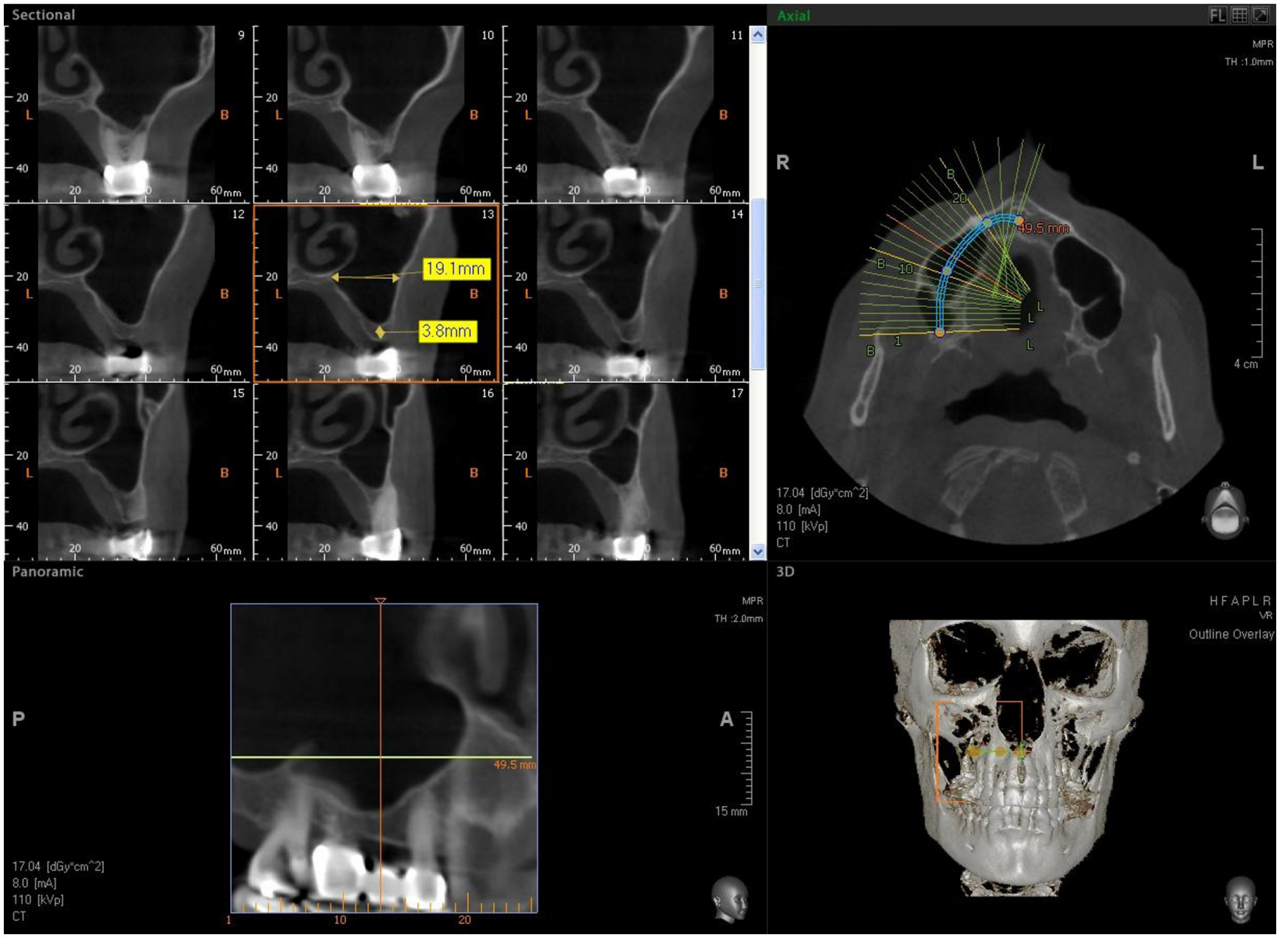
CBCT image showing reduced bone height in the sinus area with a residual alveolar ridge height between 2 and 3 mm.
